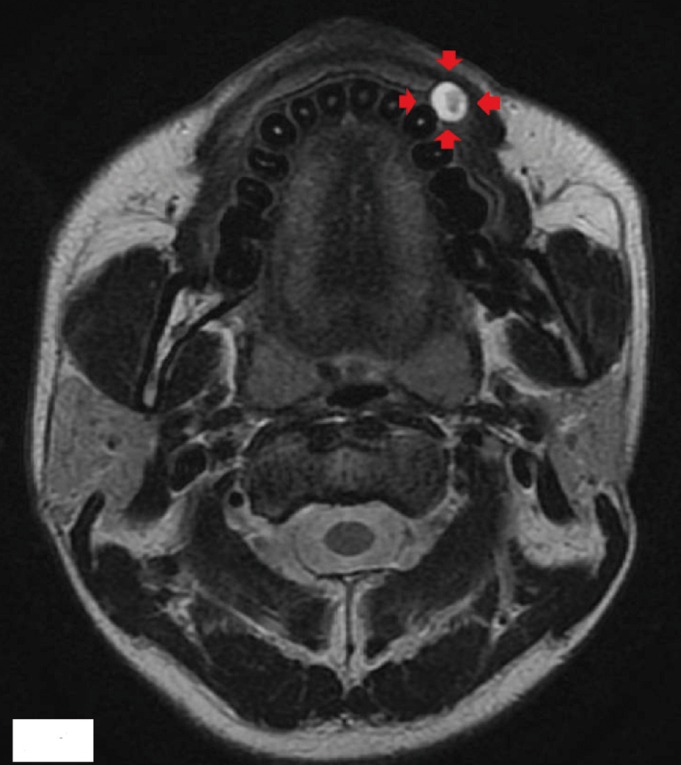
Preoperative magnetic resonance imaging, axial view. There is a 12- × 10- × 10-mm cystic lesion of the upper lip with high signal intensity on T2-weighted images. There is a clearly demarcated border between the lesion and the surrounding tissues.
